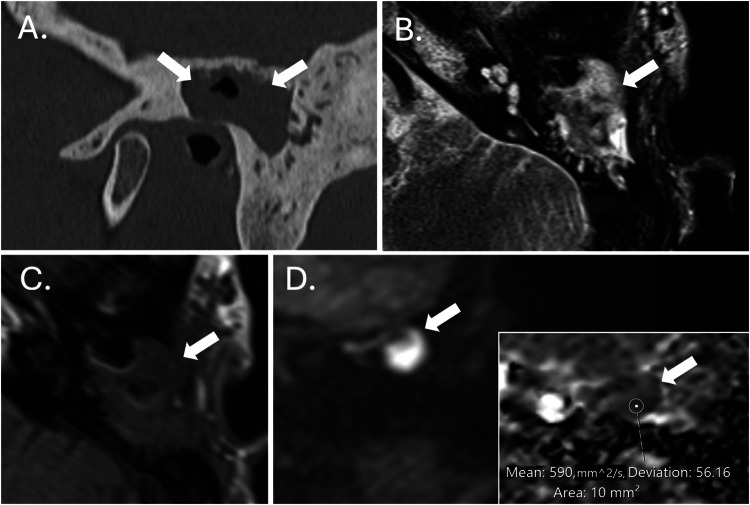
Follow-up imaging following a left CWUM. Imaging of the left temporal bone in a patient who had previously undergone a CWUM for cholesteatoma. The sagittal CT (A) shows nonspecific opacification of the mastoid cavity (arrows), which is expansile and extends above the EAC. On MRI, there is an area of increased signal on T2W (arrow on B), low to intermediate signal on T1W (arrow on C) and markedly increased signal on HASTE DWI (arrow on D) with low ADC values (590 × 10^−^^6^ mm^2^/s) (arrowed on inset image) compatible with residual cholesteatoma
